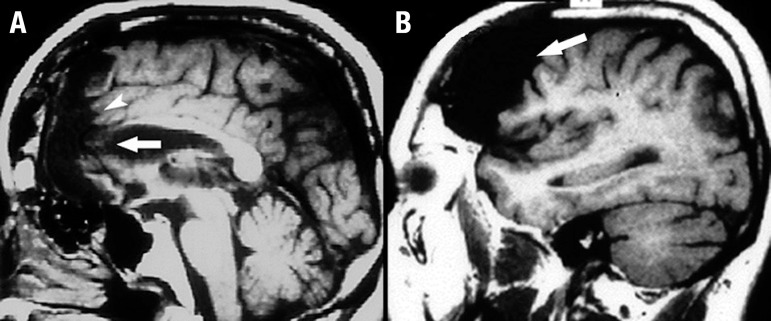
Sagittal brain MRI images. Sagittal T1-weighted images [A and B] show
